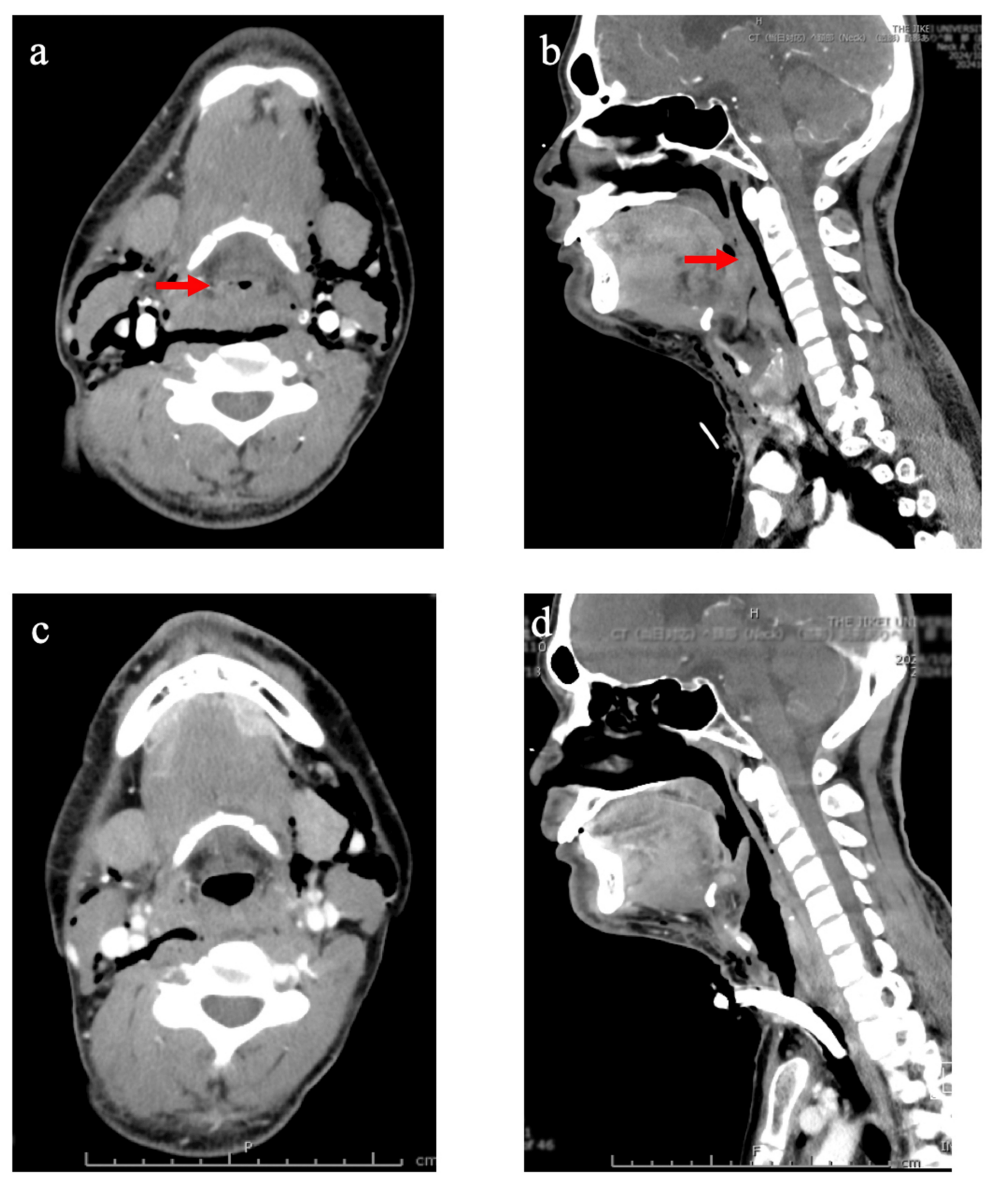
Enhanced CT images of the neck (a) Horizontal image and (b) sagittal CT image performed in the emergency room on the day of admission, and (c) horizontal CT image and (d) sagittal CT image four days after the operation. The red arrow indicates narrowing of the airway due to the hematoma. Edematous swelling suggestive of hematoma was observed in the epiglottis, bilateral aryepiglottic folds, and arytenoid regions; however, no obvious extravasation was identified. The airway was diffusely narrowed at the oropharyngeal, hypopharyngeal, and laryngeal levels (a and b). However, edematous swelling was present, but showed a trend toward improvement (c and d) CT: computed tomography
